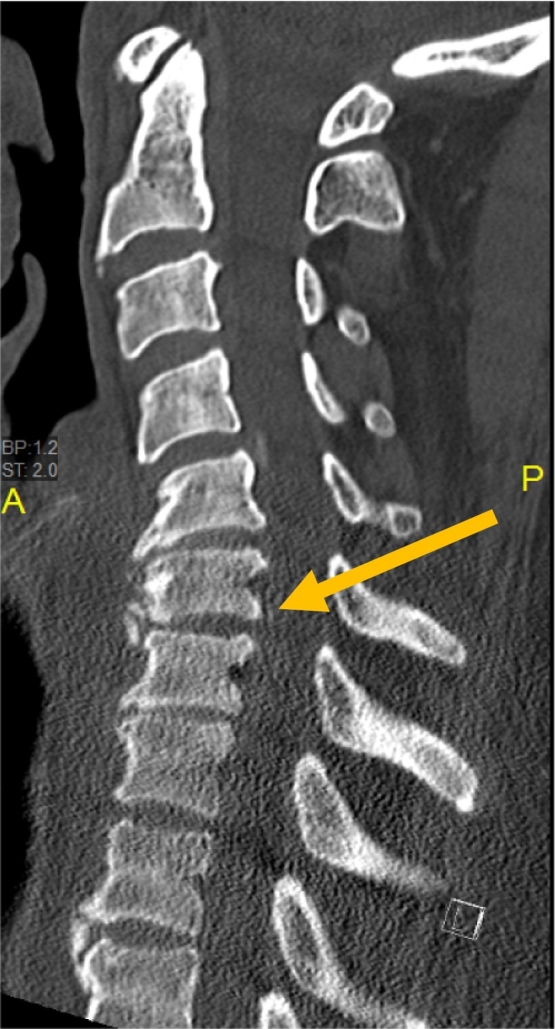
CT scan of cervical spine (sagittal view) showing no calcified posterior longitudinal ligament.
